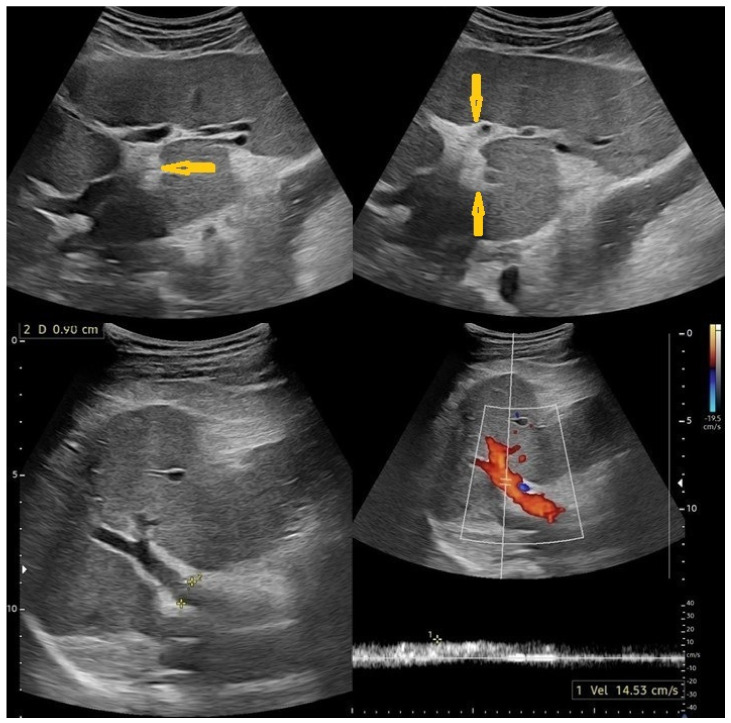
Selected sections of ultrasonography of a 40-year-old man’s liver show periportal fibrosis (arrows) of hepatic Schistosomiasis with an average liver size and normal echo patterns, no focal lesion, portal vein (PV) diameter = 9 mm with reduced flow velocity to 14 cm/s, and no prominent portosystemic collateral.
